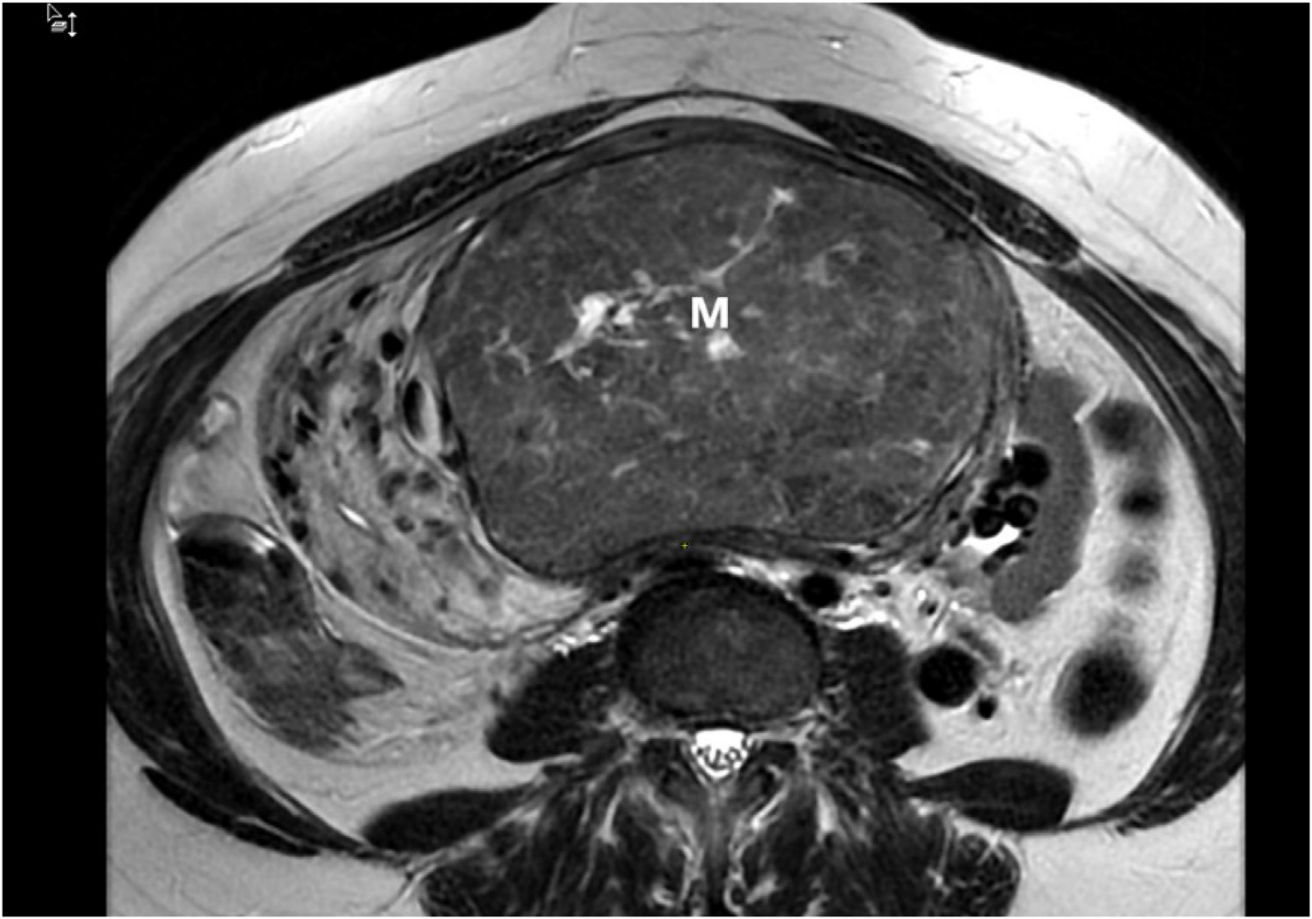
MRI image of the pelvis, axial image demonstrates a large heterogeneous exophytic mass arising from the uterus (M).
